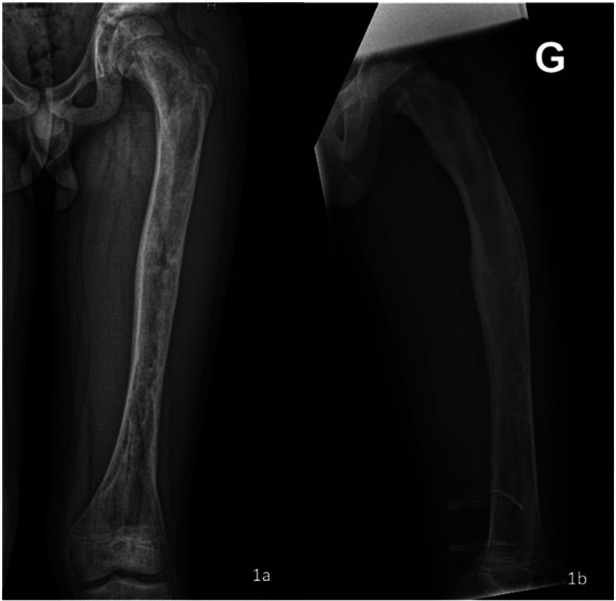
X-ray images of the left femur after removal of osteosynthetic materials: the frontal coronal plane image revealed a variety of radiolucent lesions in the diaphysis, with sclerotic edges, scalloping and a “ground-glass” appearance (a) sagittal plain radiographs showed an increased sagittal radius of curvature and retrotorsion of the proximal femur (b).
